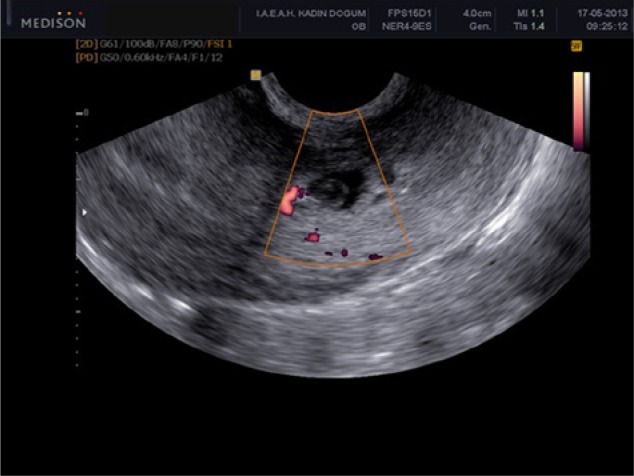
Ultrasound examination of case 1.
